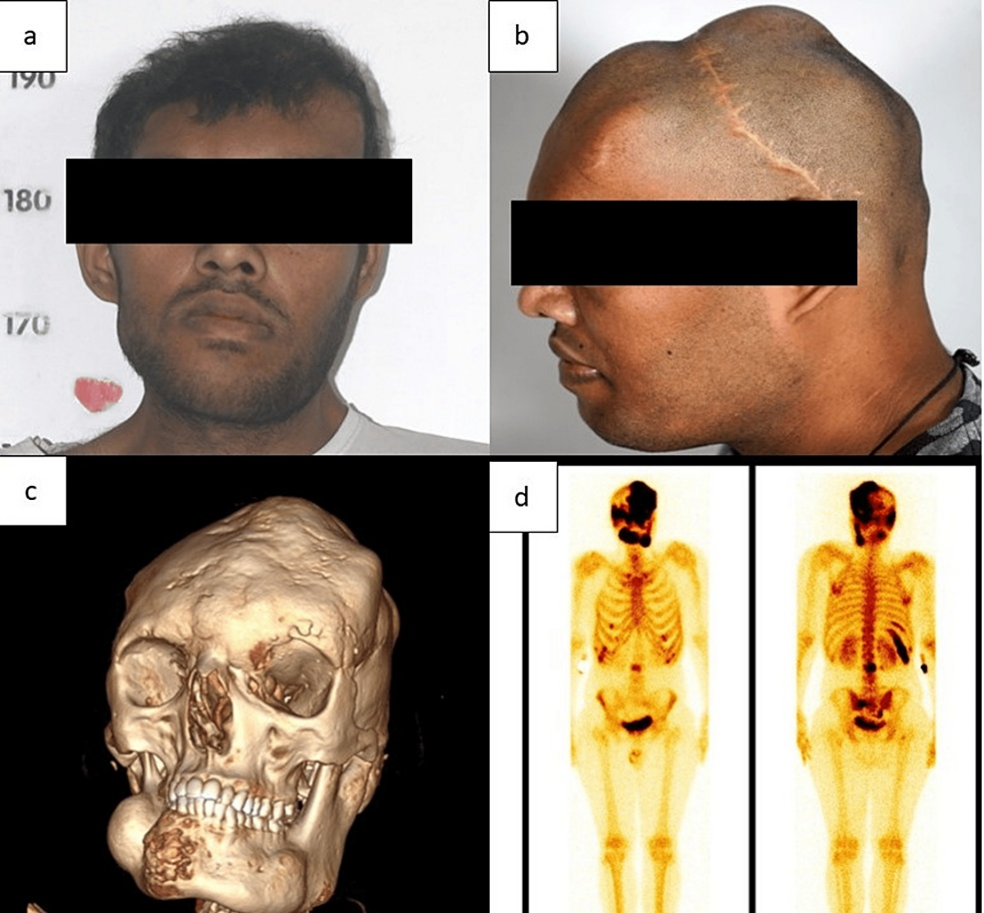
Clinical image of the patient showing acrogigantism (height 192 cm) (a) and irregular bumpy skull vault consistent with craniofacial fibrous dysplasia and acanthosis nigricans in the nape of the neck (b). Three-dimensional CT reconstruction of facial skeleton showing facial asymmetry (c) and whole body Tc99 methylene diphosphonate skeletal scintigraphy showing increased osteoblastic activity in skull bones, maxilla, both sides of the mandible, multiple bilateral ribs, L3 vertebra, and sacrum with bilateral ala suggestive of polyostotic fibrous dysplasia (d).
